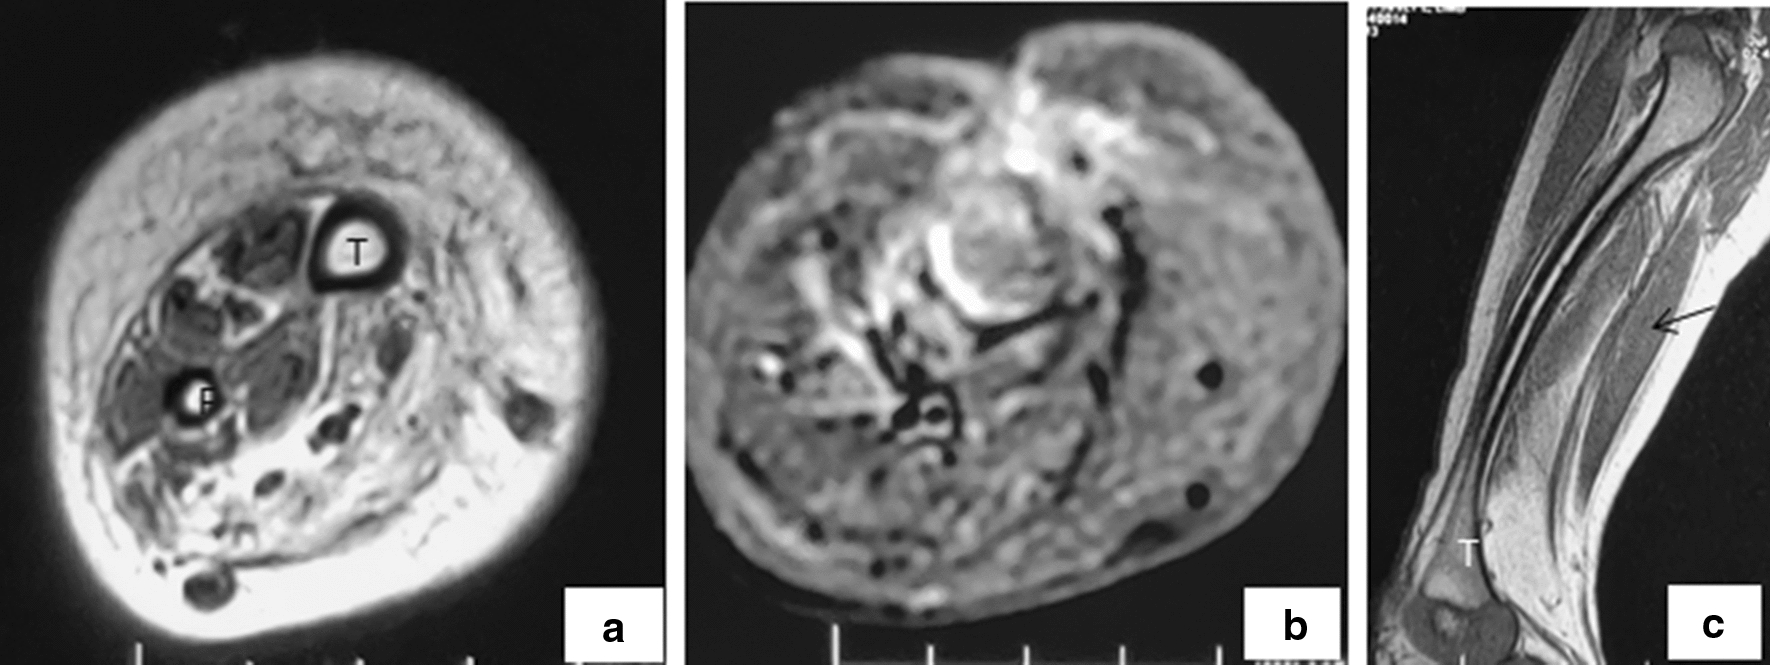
Response to treatment on MRI. Follow up MRI shows resolution of reticular hyperintense pattern on T1 (a) and STIR (b) axial images with increase in bulk of the calf muscles (c) as compared to pre-treatment scan (Fig. 2)
